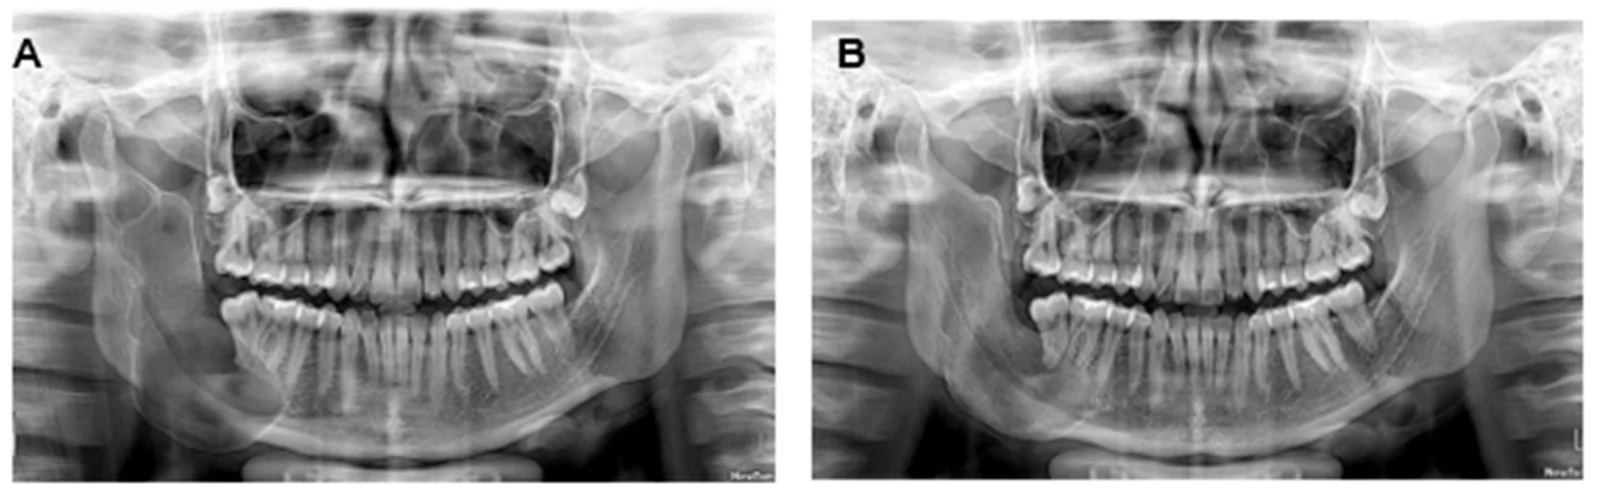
Postoperative panoramic radiographs after enucleation. (A) At 3 months and (B) 6 months after the second surgical stage, showing progressive bone healing of the surgical site without evidence of residual lesion or recurrence.
